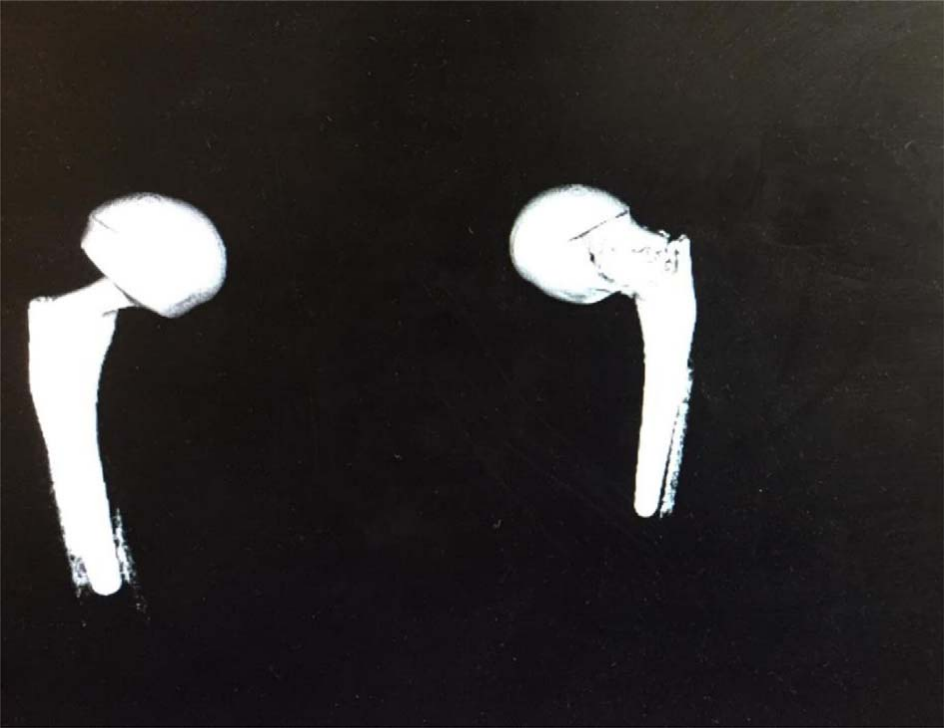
CT scan (3D Construction), showing the dislocation.
